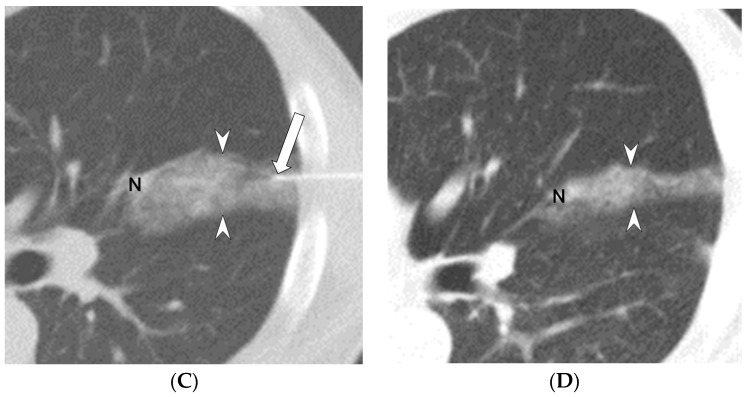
A 49-year-old man undergoing CT-guided localization with PBV dye. Preprocedural chest CT revealed a 0.87-cm part-solid nodule (N) in the left upper lobe ((A), CT axial view). Following a 4.80-cm needle insertion localization, CT images indicated that consolidation and the ground-glass opacities ((B), white arrowheads) to the left side of the nodule (N), and these findings were consistent with grade 2 pulmonary hemorrhage (B). The needle was then slowly relocated closer to the pleural area ((C), white arrow). A significant amount of pulmonary hemorrhage was observed within the patient’s body ((D), white arrowheads).
